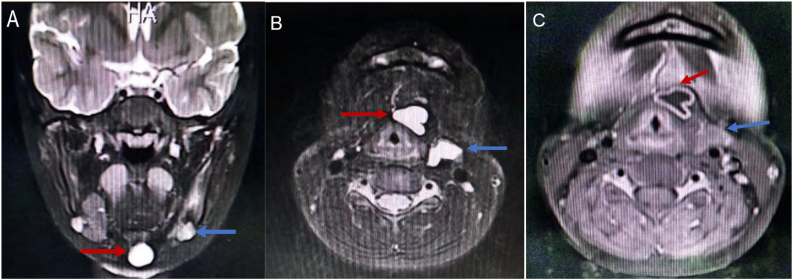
(A) Axial and (B) Coronal Magnetic Resonance Imaging (MRI) scan of the neck showed T2-weighted hyperintense signals seen between the hyoid (red arrow) and thyroid cartilage and in front of the left carotid artery (blue arrow). (C) Enhanced MR Imaging (DCE-MRI) scan of the neck showed the hyoid bone and the anterior thyroid cartilage lesions showed ring enhancement (red arrow) and the lesions in the left anterior carotid artery showed mild uneven enhancement (blue arrow).
